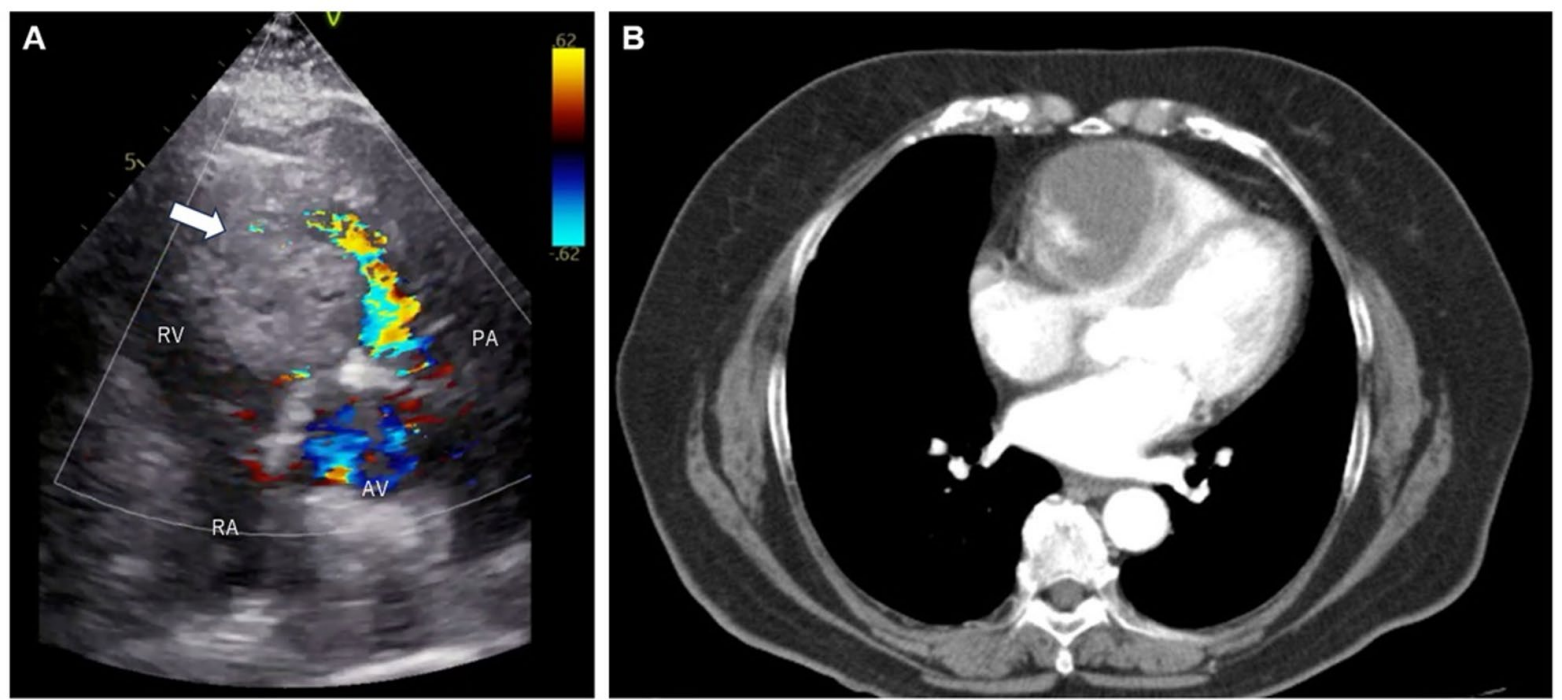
Preoperative imaging findings. (a) Transthoracic echocardiography revealed a right ventricular mass approximately 6 cm in diameter (white arrow), with mosaic flow indicating right ventricular outflow tract stenosis. (b) Contrast-enhanced CT demonstrated a heterogeneously enhancing mass, with contrast uptake extending from the periphery toward the center. RV, right ventricle; RA, right atrium; AV, aortic valve; PA, pulmonary artery
